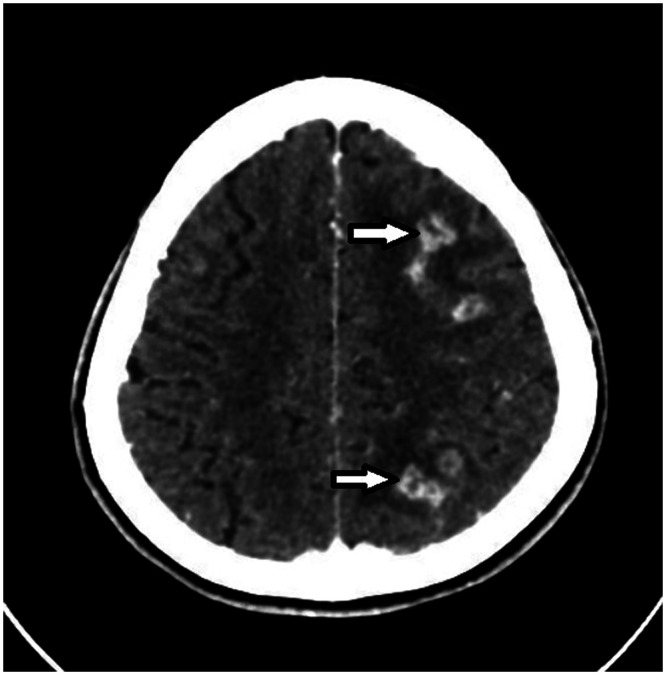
A computed tomography head scan with contrast showing multiple cortical/subcortical ring-enhancing lesions in the frontoparietal lobe with surrounding vasogenic edema (arrows).
